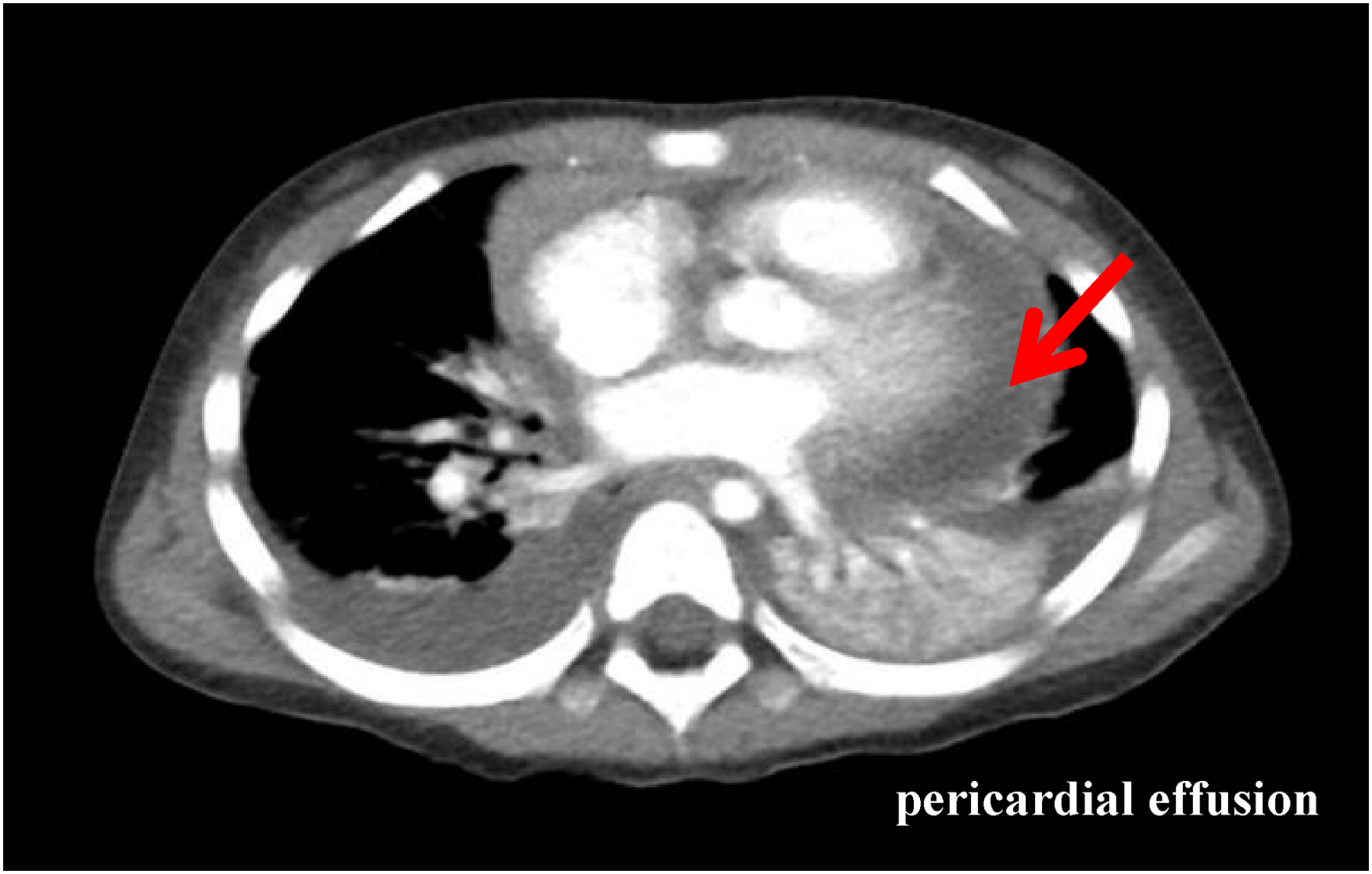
Enhanced chest CT (the third day of admission): bilateral lung infection with massive consolidation; enlarged heart, moderate pericardial effusion, pericardial thickening, and enhancement; and a small amount of bilateral pleural effusion.
